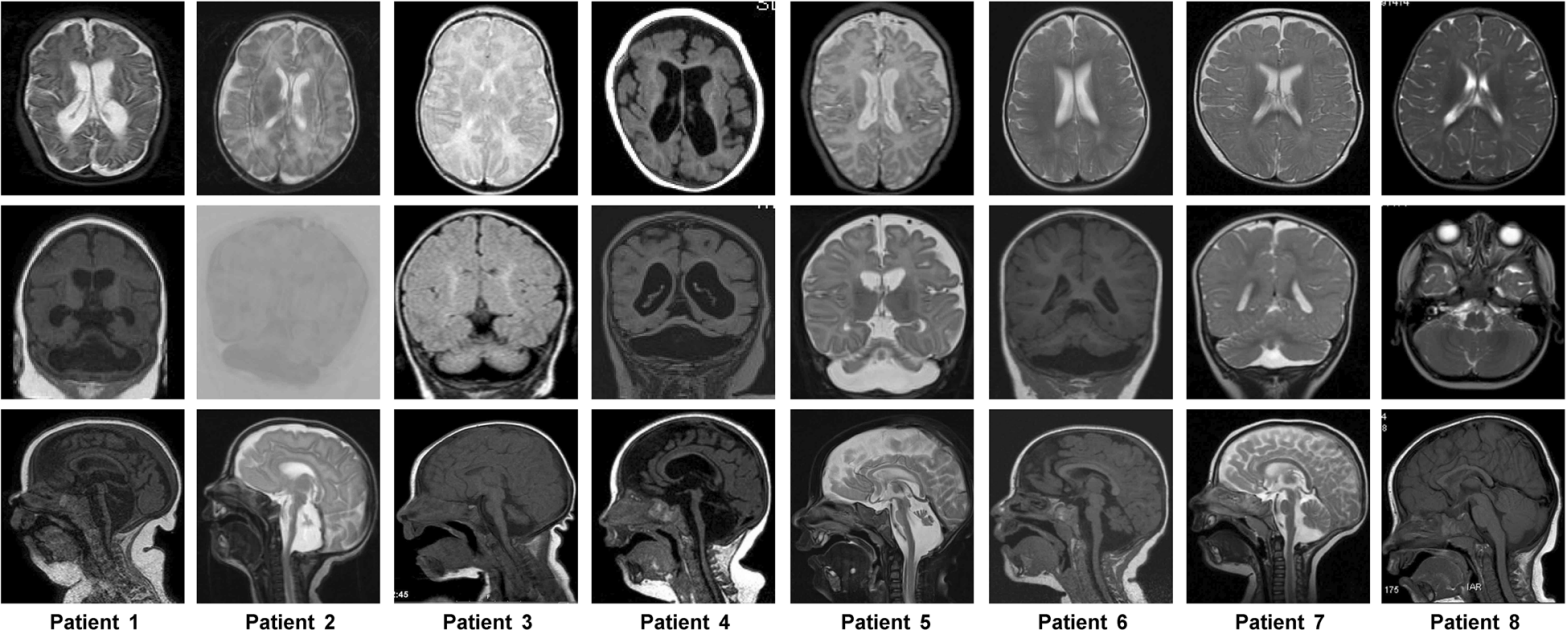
Selected axial, coronal and sagittal MR images from eight male individuals with a *CASK* alteration. The coronal images (second row) show hypoplastic, flattened cerebellar hemispheres with proportionally reduced size of the vermis in patients 1–7. The sagittal images in the third row show intact corpus callosum in all cases, low forehead indicative for microcephaly and pontine hypoplasia in patients 1–7. Pontocerebellar hypoplasia is severe in patients 1–5, moderate in patient 6, and mild in patient 7. Cerebellum and pons of patient 8 are normal. A mildly reduced number and complexity of the frontal gyri are seen in patients 1 and 7, and cortical atrophy in patients 1 and 4 (axial images in first row). MR imaging was performed at the age of 6 months (patient 1), 5 months (patient 2), 5 years (patient 3), 2 months (patient 4), 4 months (patient 5), 11 months (patient 6), 10 months (patient 7), and 16 months (patient 8).
